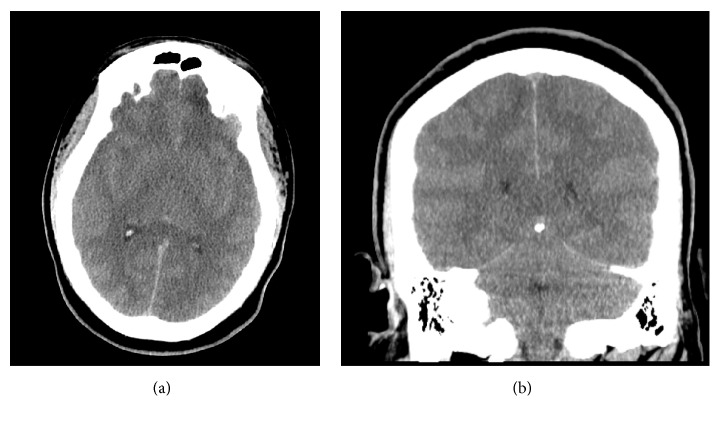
Axial cut (a) and coronal cut (b) of the noncontrast computed tomography (CT) of the head of the patient after becoming obtunded with nonreactive pupils. The images show diffuse cerebral edema with compression of lateral ventricles, effacement of the sulci, and obliteration of the perimesencephalic cisterns.
